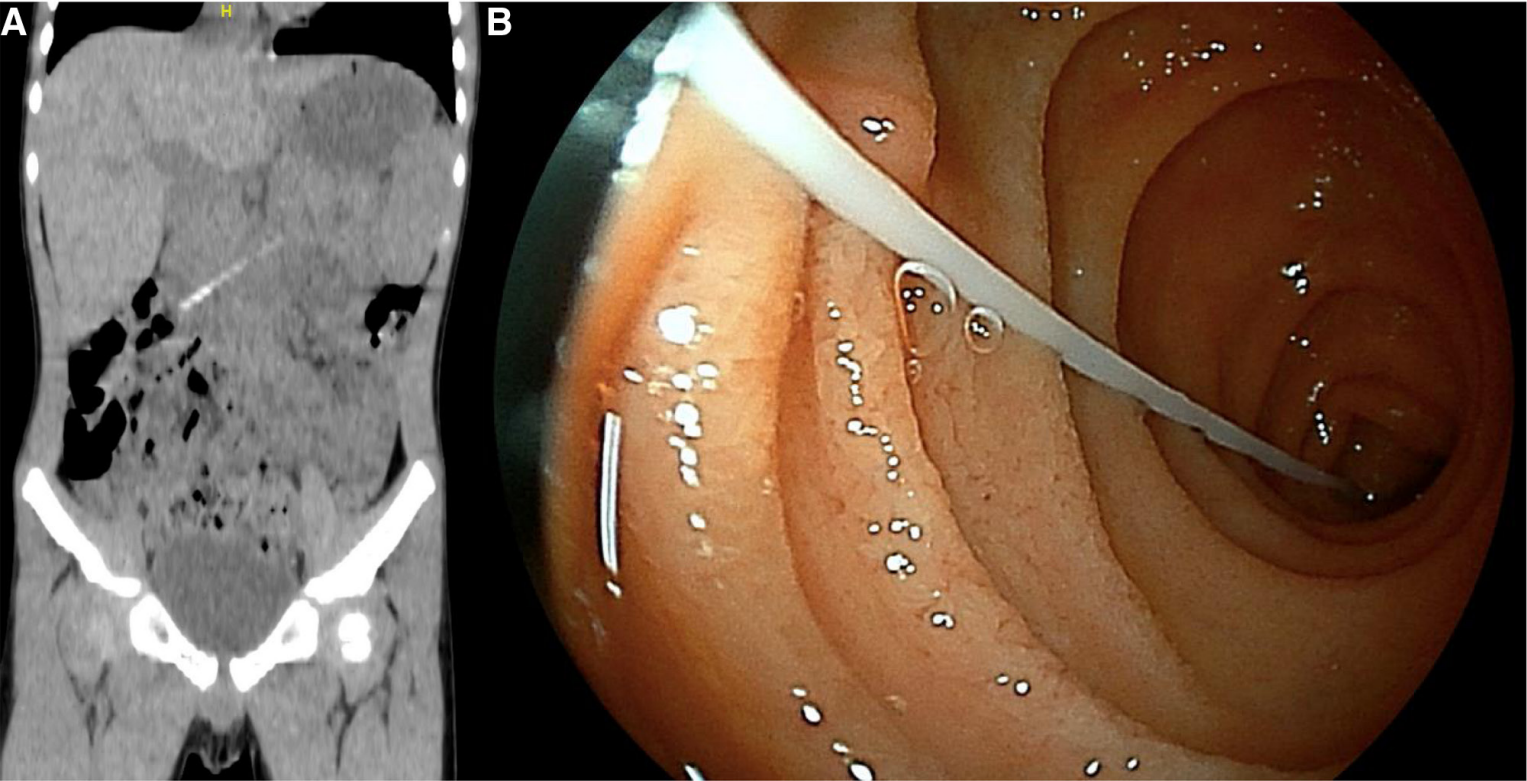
Nasal swab retained in the third portion of the duodenum. A) Computed tomography 5 d after the accidental ingestion. B) Endoscopic image. The top of the nasal swab was on the anorectal side. Only mild redness was seen on the contact surface.
